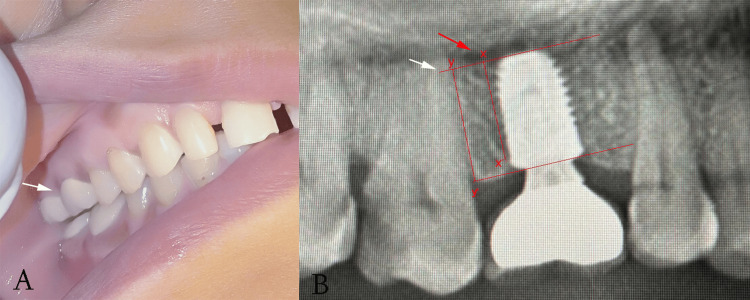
Radiographic findings. Dental implant at the maxillary right first molar showing (A) the clinical position of the gingival margin (white arrow) and (B) measurement of implant length (white arrow) and marginal bone level (red arrow). y-y' : implant length (in mm), x-x' : marginal bone height (in mm) Image analysis was performed using ImageJ software (Version 1.54, National Institutes of Health, Bethesda, MD, USA). All measurements were obtained after image calibration and recorded in the designated units of measurement.
